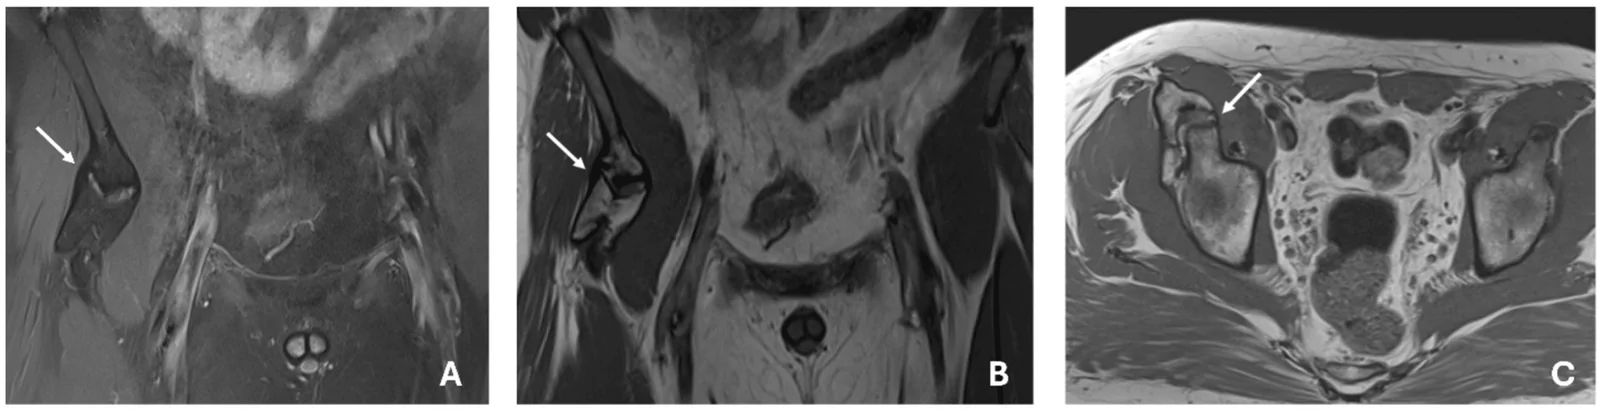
T2 coronal fat-saturated (A), T1 coronal (B), and T1 axial (C) MRI images confirming the bony fragment projecting from the right anterior inferior iliac spine with pseudoarthrosis, pelvic digit MRI: magnetic resonance imaging
